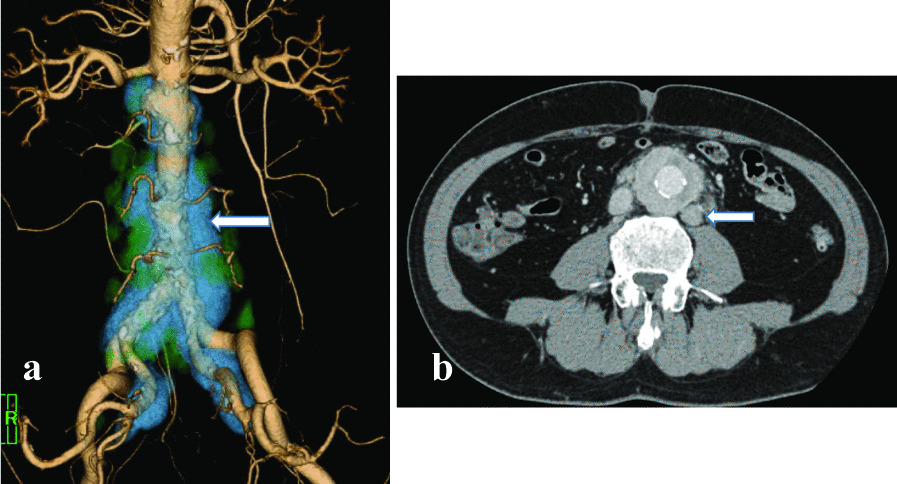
Abdominal enhanced CT revealed a soft tissue mass around the bilateral common iliac arteries from the abdominal aorta (a white arrow, 3D-CT). Additionally, multiple lymphadenopathy is observed around the abdominal aorta (b white arrow)
